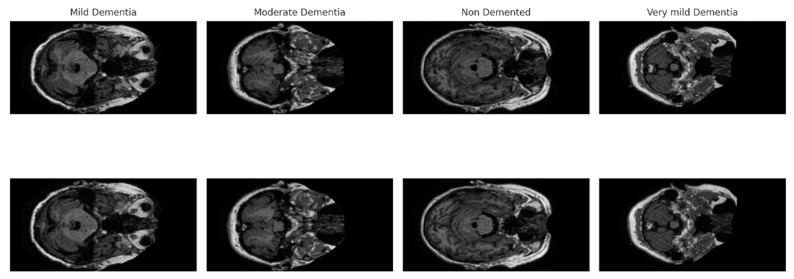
Example images of classes in the OASIS MRI dataset.
